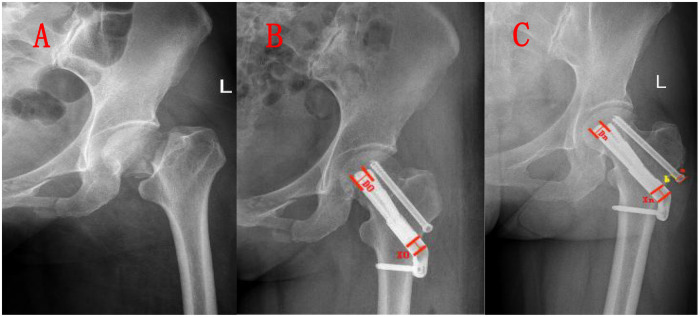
A 55-year-old male patient with a garden type IV left femoral neck fracture caused by a traffic injury. The fracture was complete with displacement of the femoral head. After comprehensive examinations, open reduction and internal fixation with FNS combined with a cannulated screw were performed on the fifth day after the fracture. A. Admission x-ray showing a complete fracture with coxa vara and inferior displacement of the femoral head. B. Immediate postoperative x-ray after fixation with FNS combined with a cannulated screw, demonstrating acceptable fracture reduction, clear fracture margins, and centrally positioned internal fixation. C. Follow-up x-ray at 15 months postoperatively, showing nearly disappeared fracture lines, excellent fracture healing, and normal hip function in the patient. a: screw cap thickness; b: exposed screw length; c: actual thickness of the screw cap.
